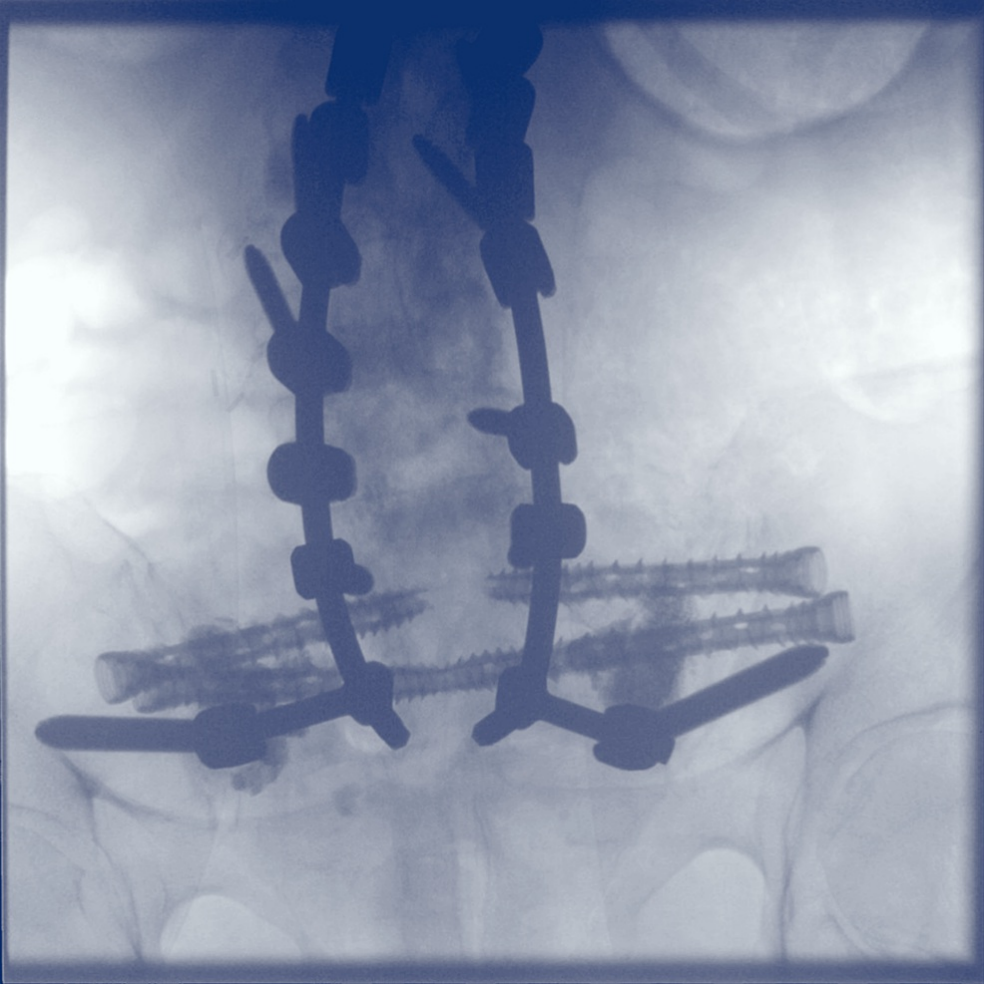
X-ray taken intraoperatively demonstrating placement of percutaneous screws spanning the sacroiliac joint and fracture lines.
